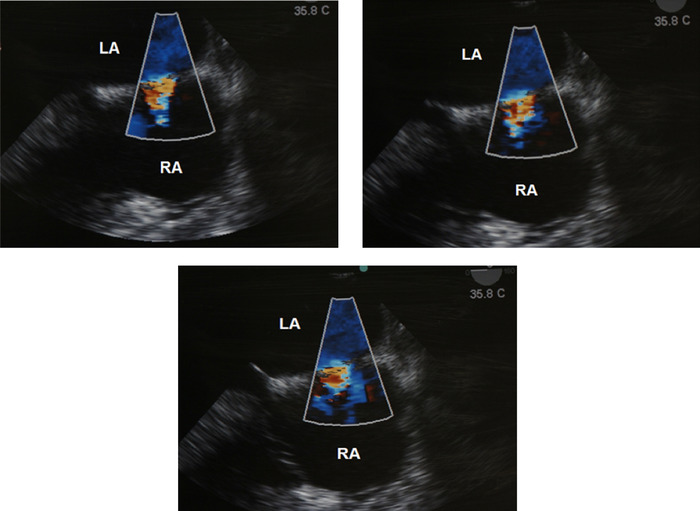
Color-flow mapping Doppler images of transthoracic echocardiography show diastolic intra-atrial jet through the atrial septal defect. Flow toward the transducer is red, flow away from the transducer is blue, and higher velocities are shown in lighter shades. Turbulent flow is indicated by green. (A color image is available online at https://doi.org/10.31486/toj.19.0119.) LA, left atrium; RA, right atrium.
